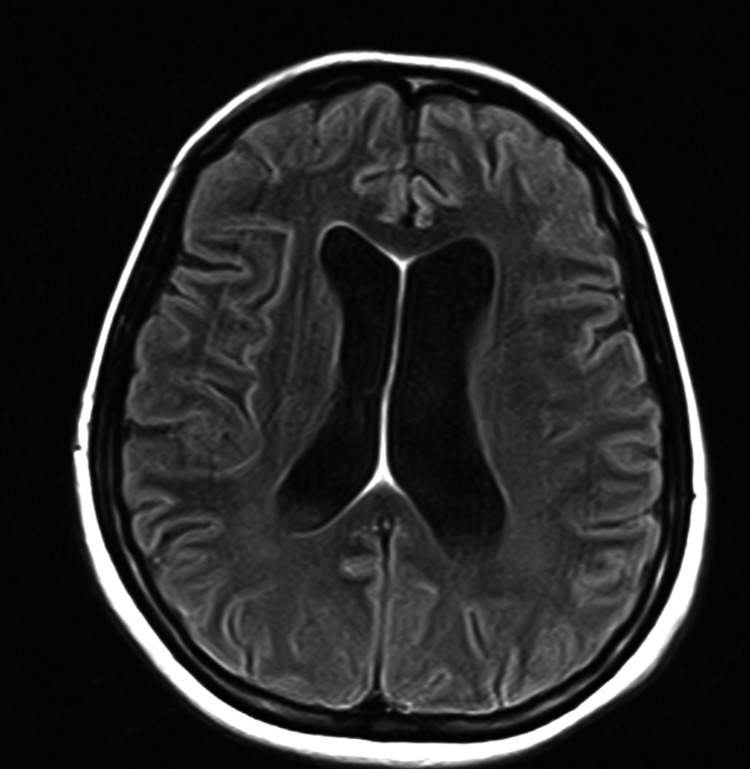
Brain MRI (axial T2 FLAIR) Axial T2 FLAIR MRI of the brain demonstrating ventriculomegaly and minimal periventricular white matter changes consistent with chronic ischemia. No acute infarction, hemorrhage, mass effect, or midline shift. Abbreviations: MRI: magnetic resonance imaging; FLAIR: fluid-attenuated inversion recovery
